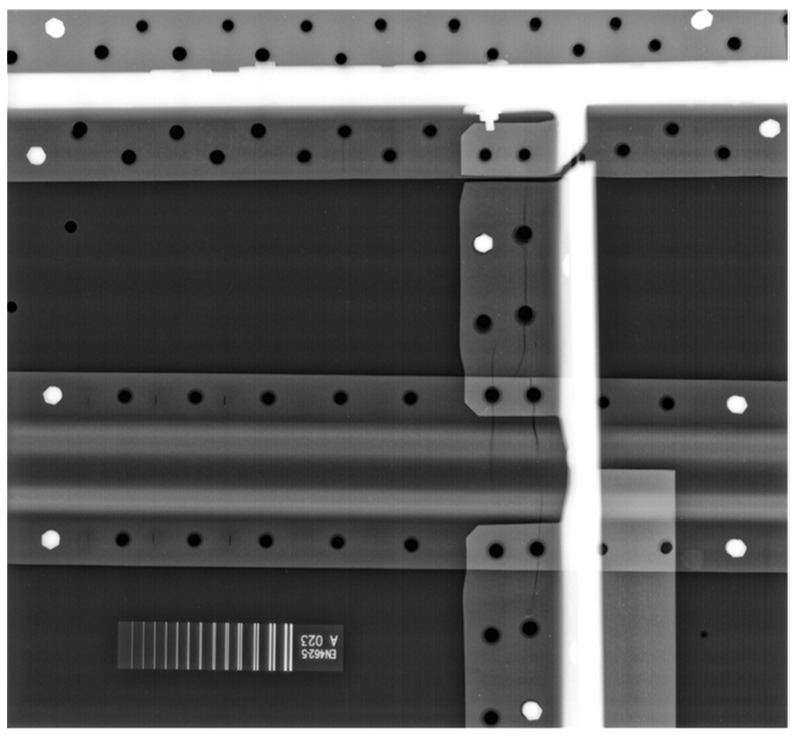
X-ray image of the airplane sample used in the initial experiments.
